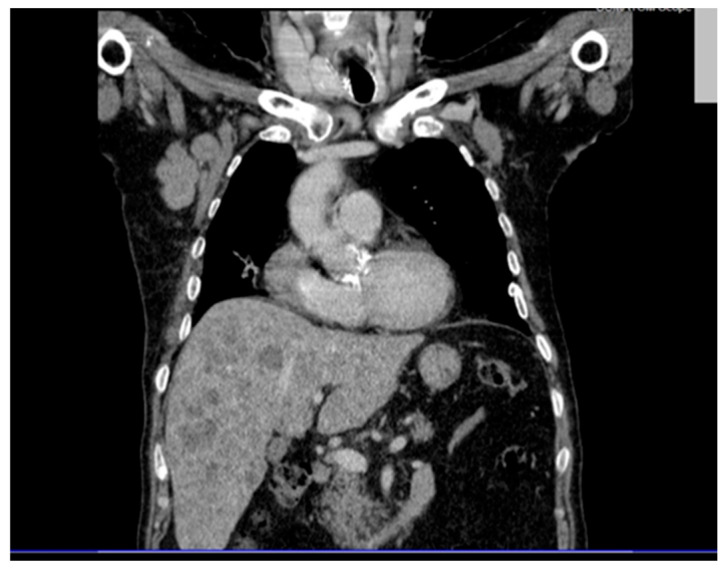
Contrast-enhanced thoracic CT scan showing pleural thickening up to 9 mm in the posterior segment of the right lower lobe and along the right oblique fissure, as well as a linear atelectasis in the middle lobe. Enlarged right axillary lymph nodes are also noted, measuring up to 32 × 41 mm.
